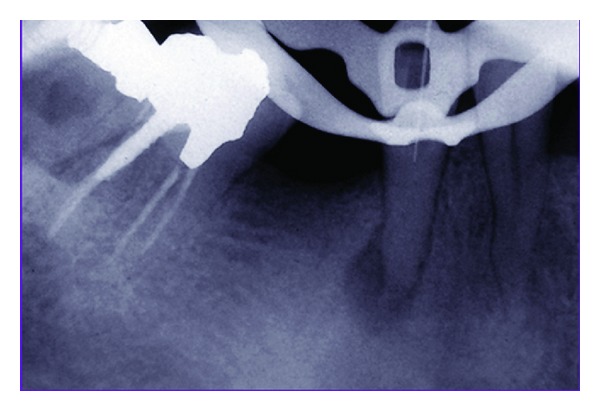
In order to find root canal's orifice, some working radiographs were taken, but root canal negotiation was impossible.
